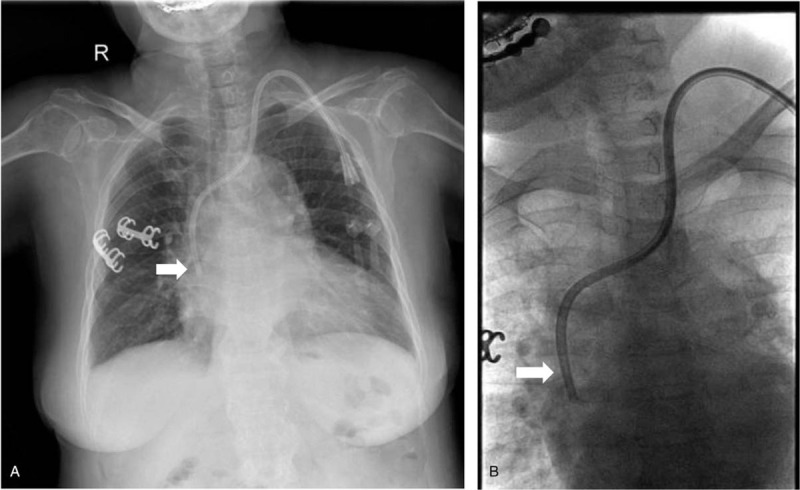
Analysis of catheter tip re-position. (A) Chest radiograph showing catheter tip has reached the correct position in the cavoatrial junction after being modulated under DSA (arrow). (B) DSA shows the hemodialysis catheter after redeployed within the right atrium (arrow). DSA = digital subtraction angiography.
